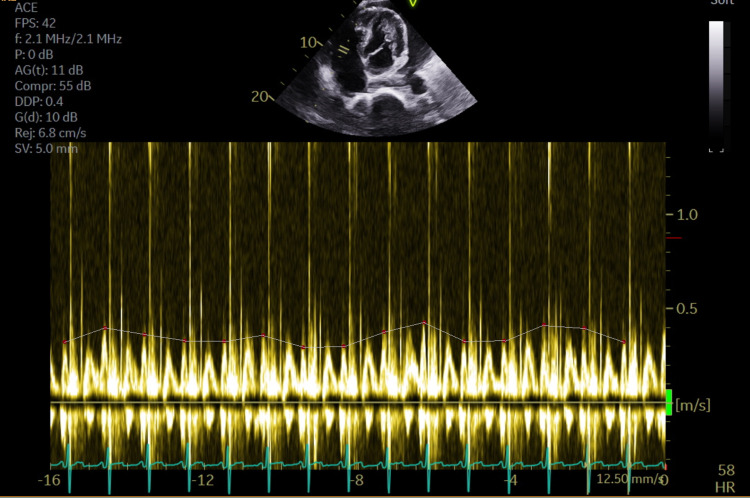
Transthoracic echocardiogram (TTE) doppler Images showing > 40% tricuspid inflow respiratory variation. The grey line has been drawn for visual appreciation.
